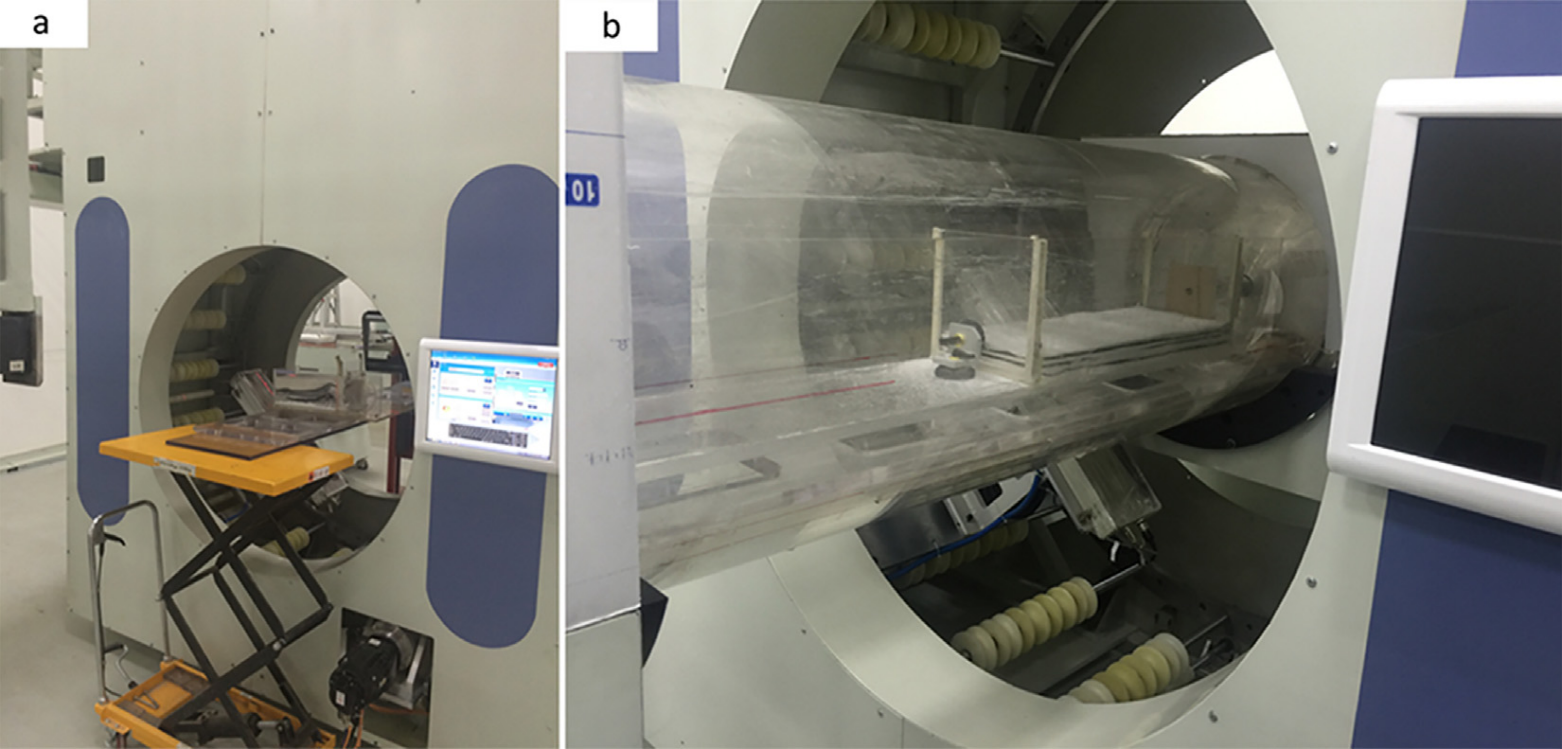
(a) The finished consecutive short-scan CT system; (b) In-situ CT scanning of an analog model in extrusion process.
